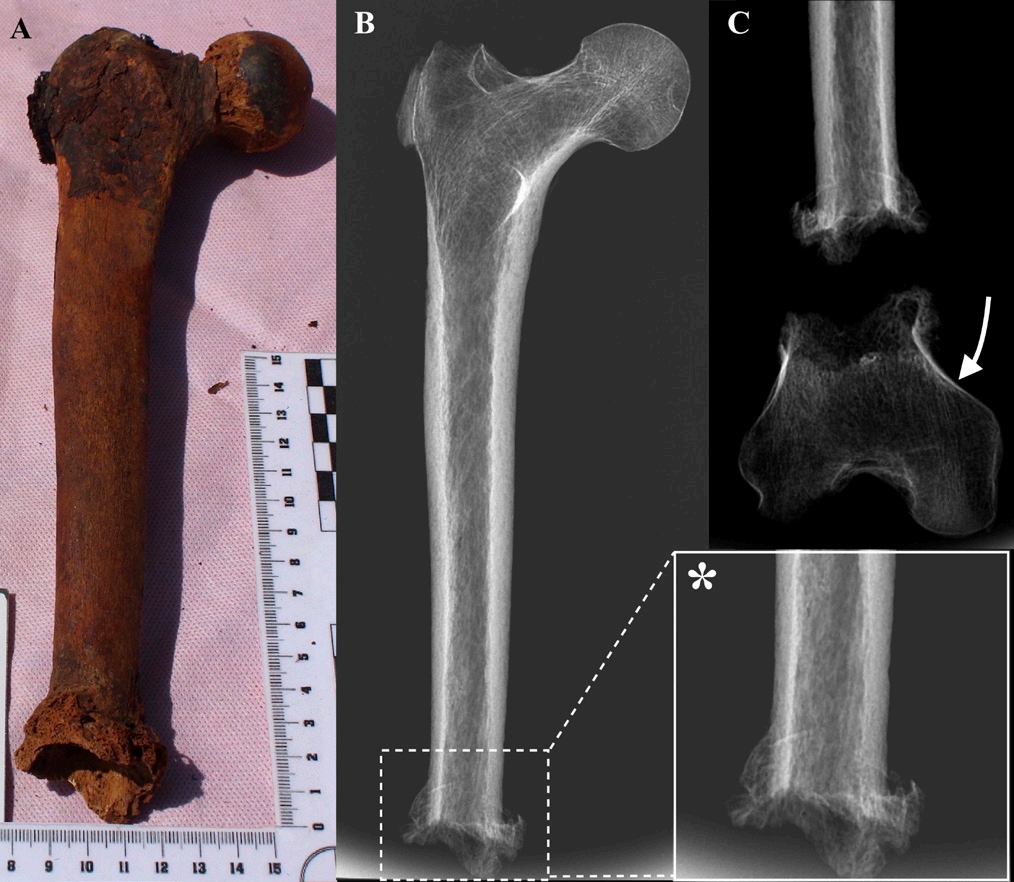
Frontal view of the right femur (on the left, (A)) and radiographic imaging (in the middle, (B)); note the mid-diaphyseal bone interruption, with slightly radiopaque bone callus visible as osseous spurs with circumferential and proximal directions (arrow). On the right (C), frontal radiograph of the right femur and distal femoral fragment found among the commingled remains (curved arrow). A transverse bone interruption is seen at the level of distal metaphysis with radiopaque bone callus, in a less extensive extent compared to the distal femoral diaphysis.
